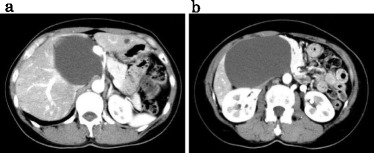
a, b Computed tomography images showing a large cystic mass between the hilar region and anterior segment of the liver without septa
